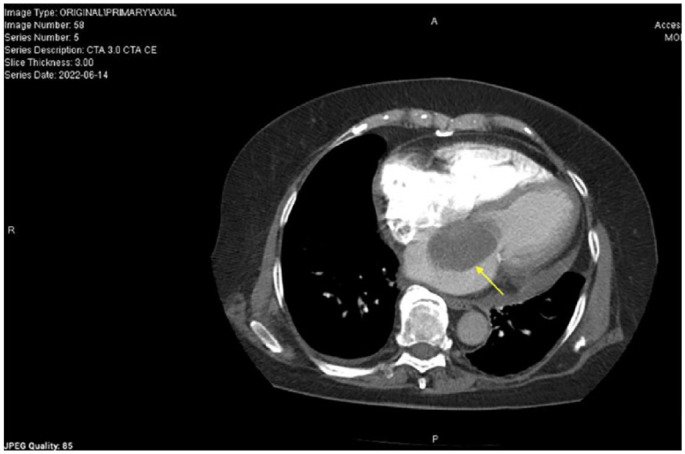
Computed tomography angiography chest of left atrial myxoma (yellow arrow).
